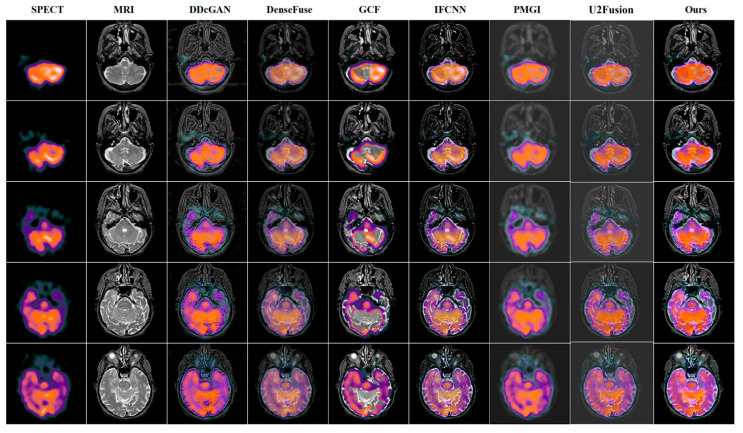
Fusion result of SPECT-MRI.
